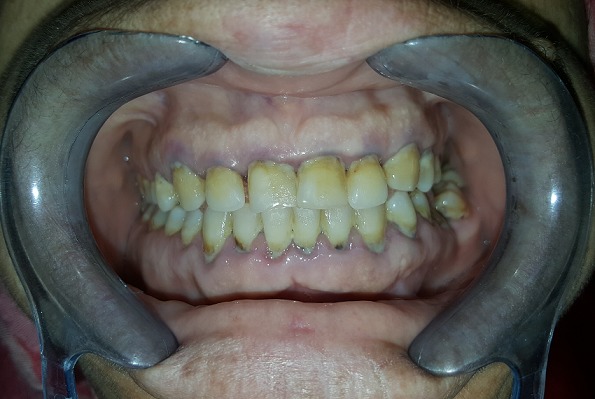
Orthopantomogram showing a generalized moderate bone loss, root fragment of 36 and hypercemoentosis (16,27,37 and 46)
